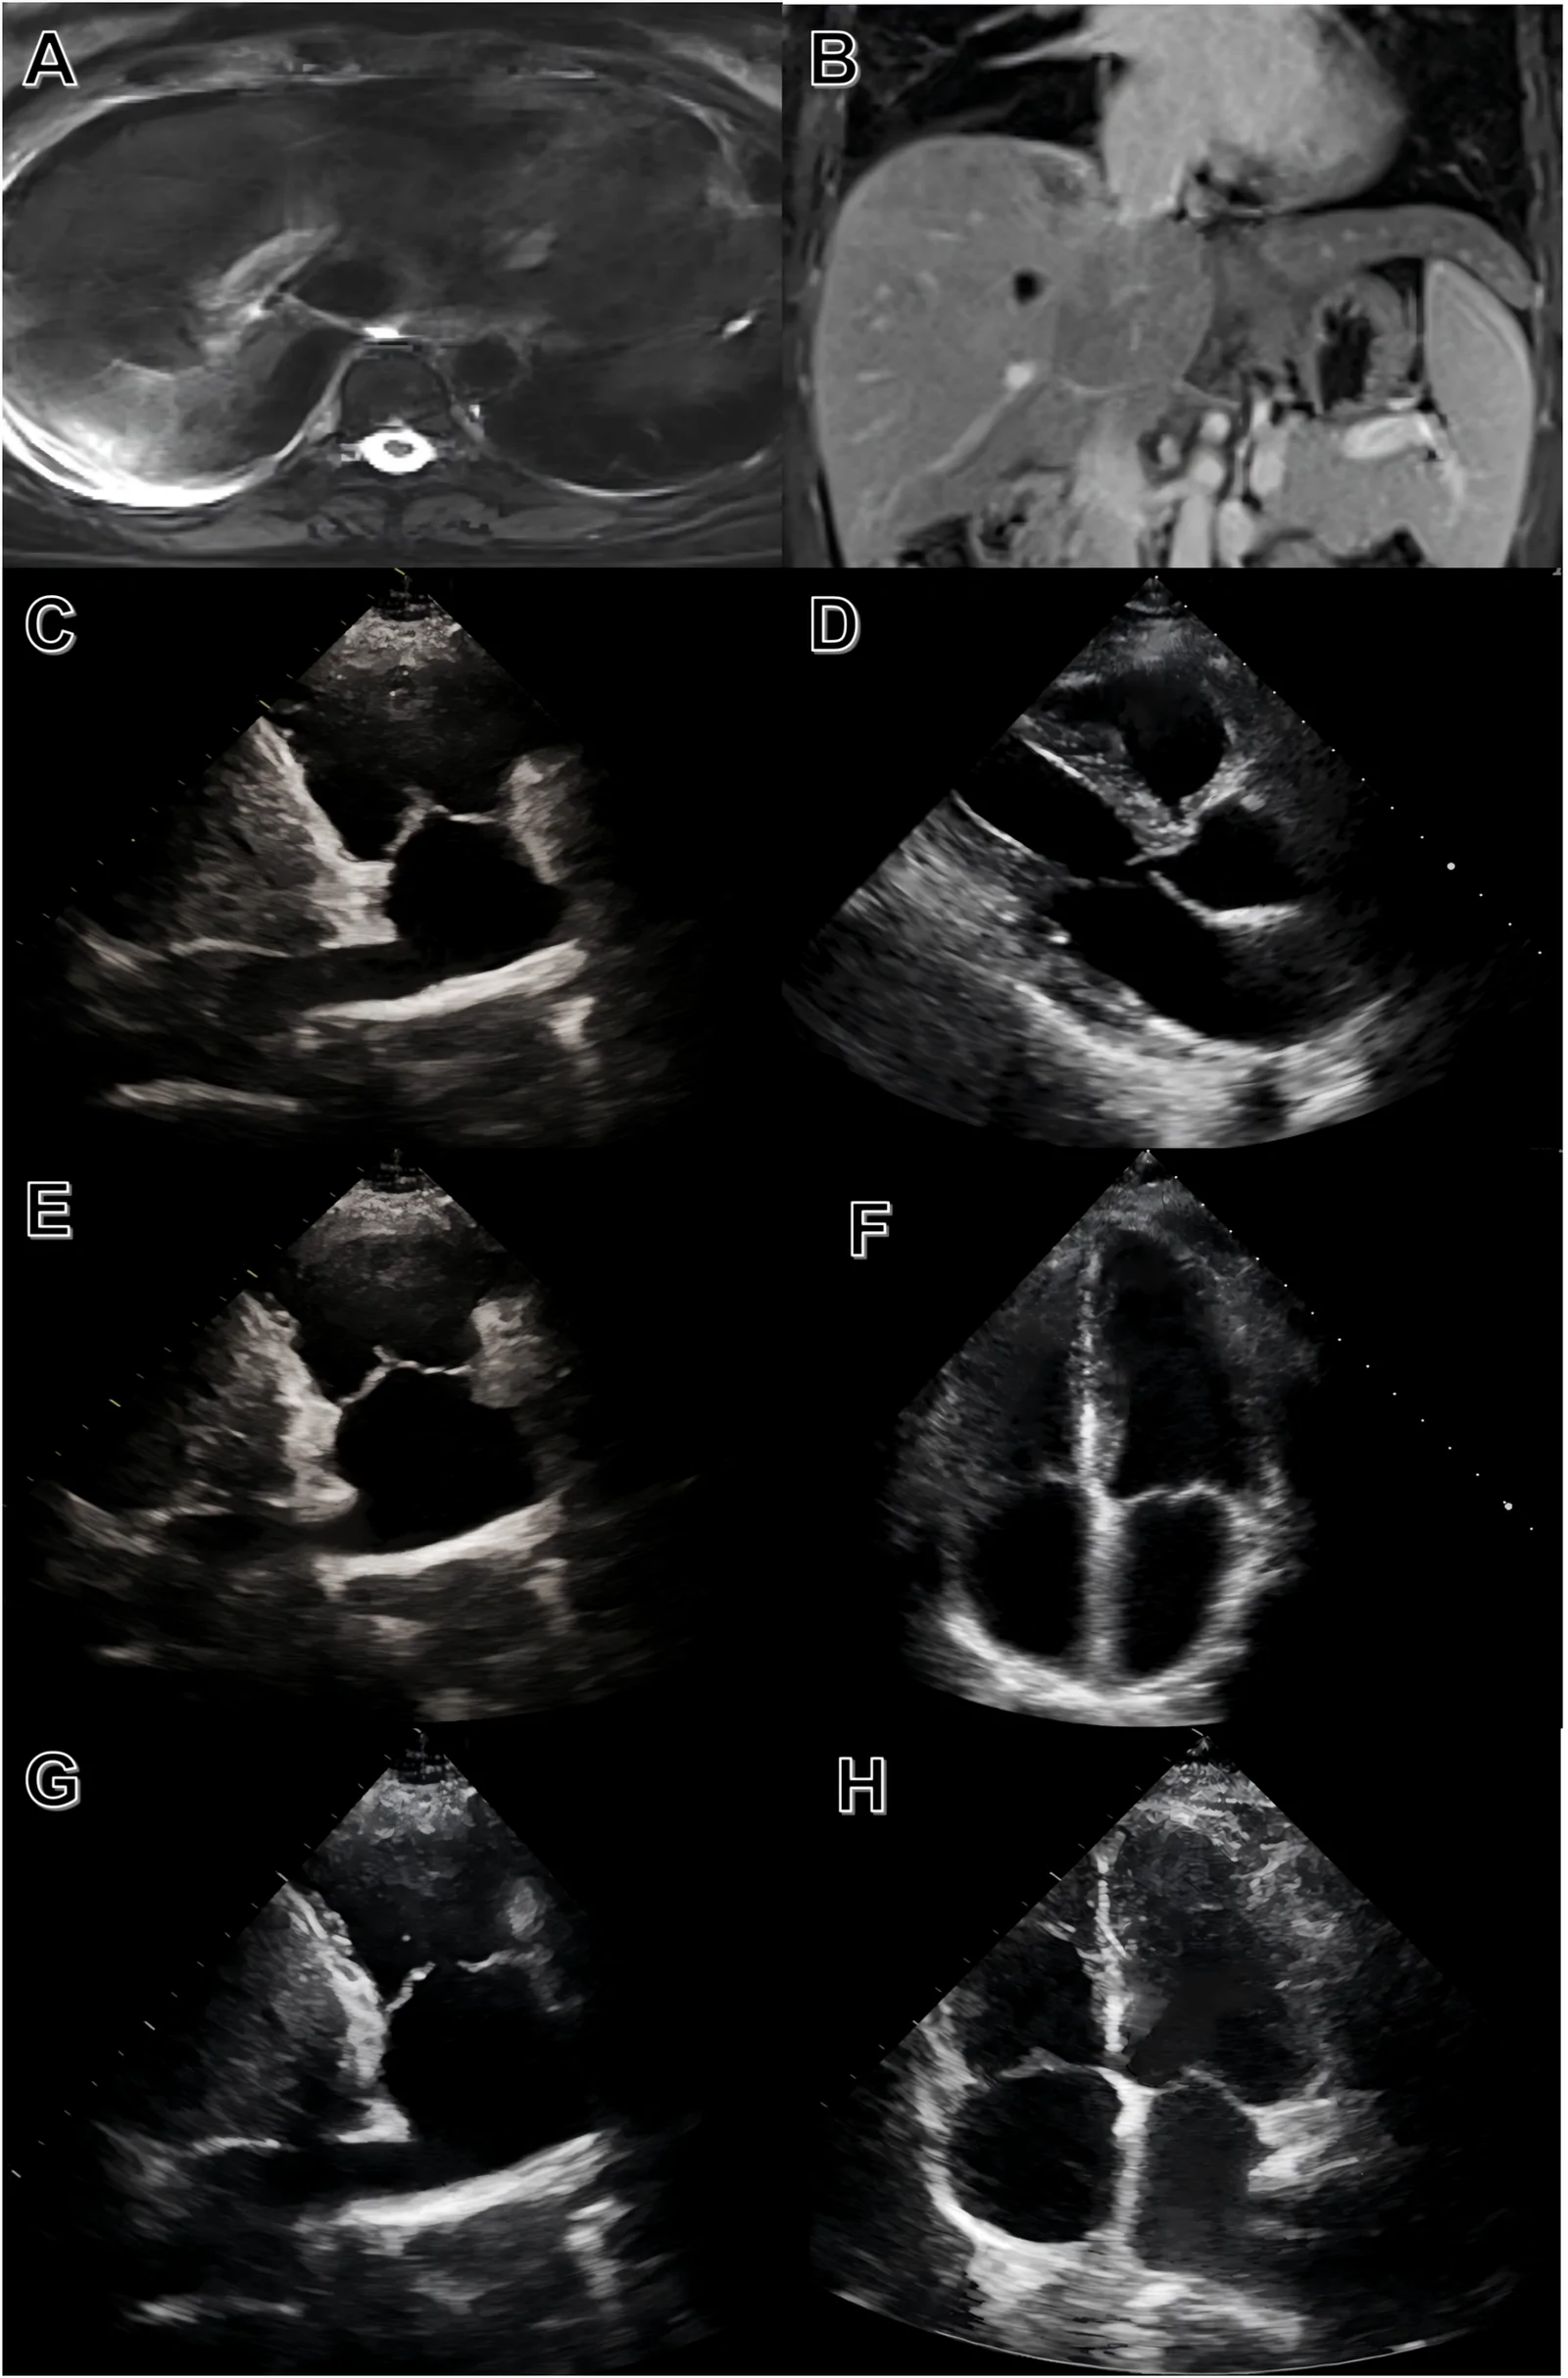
Postoperative follow-up images. (A, B) abdominal magnetic resonance images obtained on January 13, 2026. (A) T1-weighted axial contrast-enhanced (T1-Ax-C+) images. (B) T1-weighted coronal contrast-enhanced (T1-Cor-C+) images. (C, D) echocardiographic images obtained on February 3, 2026. (E, F) echocardiographic images obtained on March 17, 2026. (G, H) echocardiographic images obtained on April 28, 2026.
